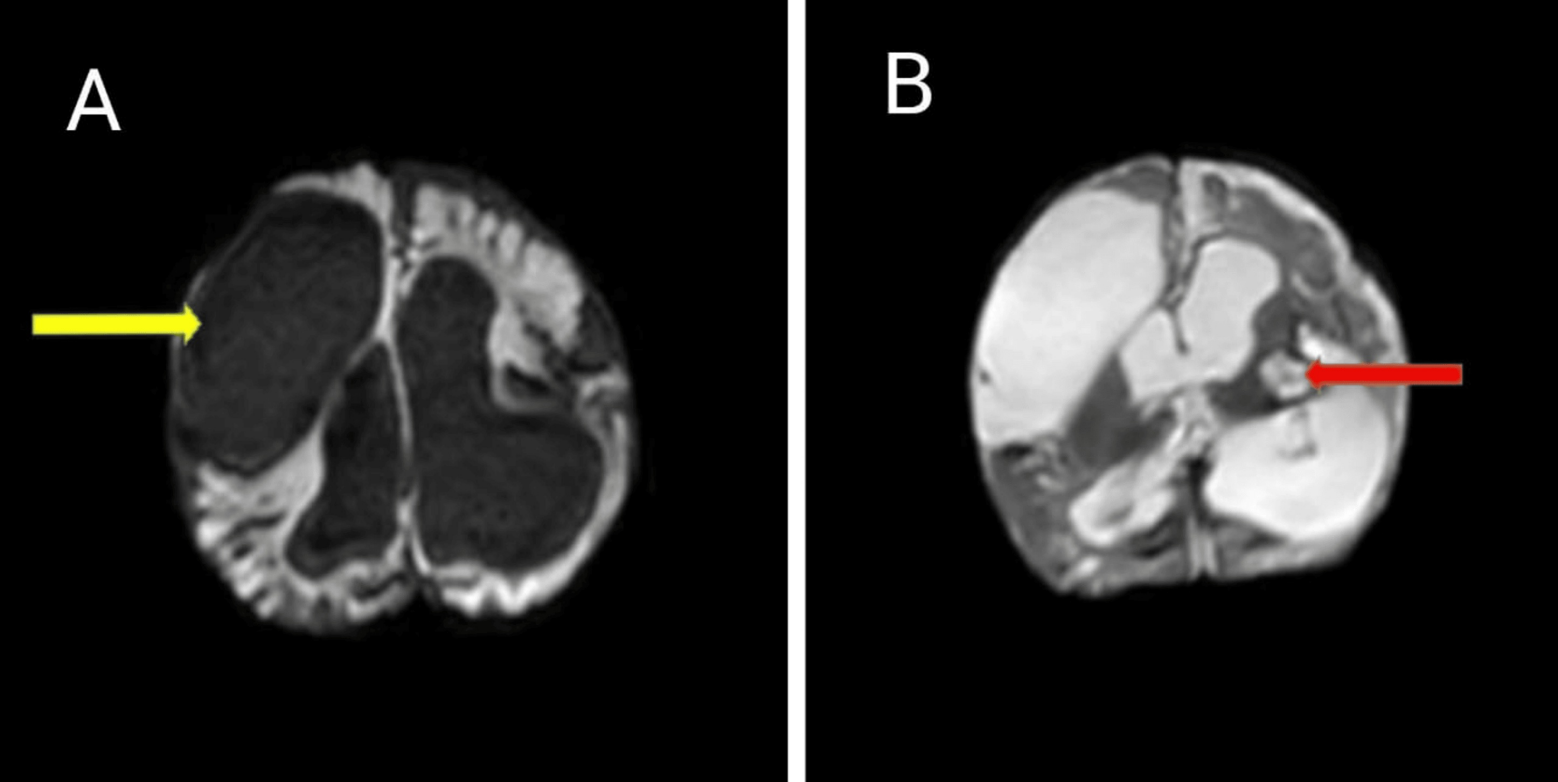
Follow up MRI at 6 months of age revealed. (A) cystic encephalomalacia (yellow arrow), (B) Chronic intraparenchymal hematoma in left thalamo capsular location with intraventricular extension into 3rd Ventricle (red arrow).
